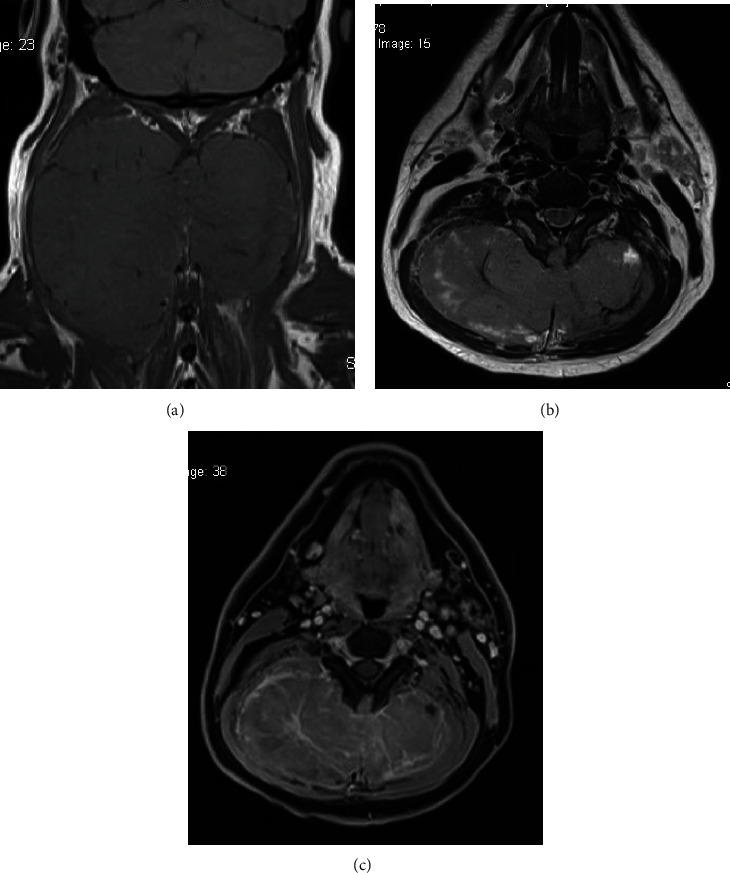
Cervical MRI: (a) coronal T1-weighted MRI and (b) axial T2-weighted MRI imaging of the cervical spine showing a posterior neck bilobed soft tissue mass showing heterogenous intermediate signal on T1 & T2 (c) axial slice of a preoperative T1 postgadolinium contrast showing patchy and reticular post contrast enhancement. MRI, magnetic resonance imaging.
